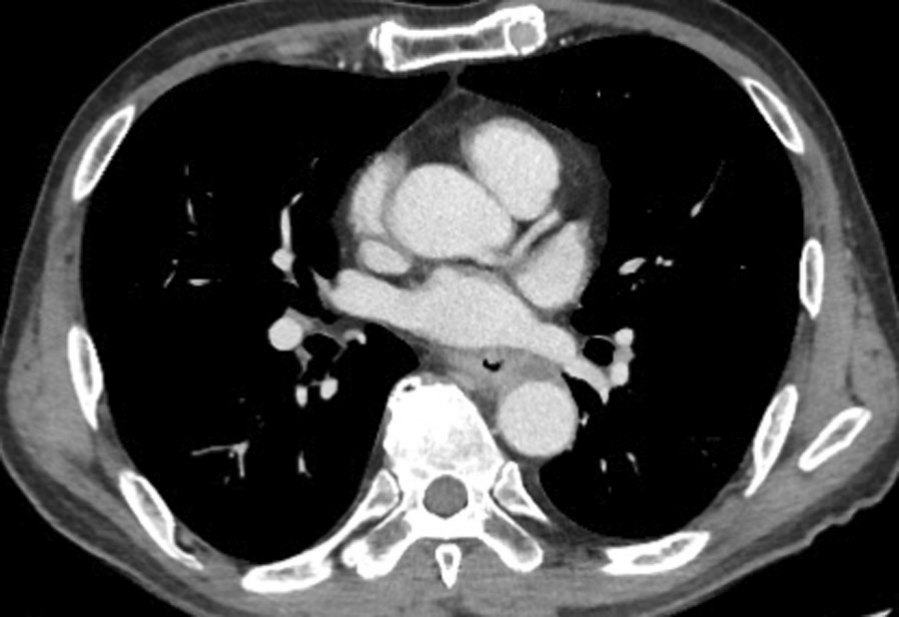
Computed tomography after neoadjuvant chemotherapy showed the esophageal tumor and the lymph node adjacent to the tumor were reduced in size
